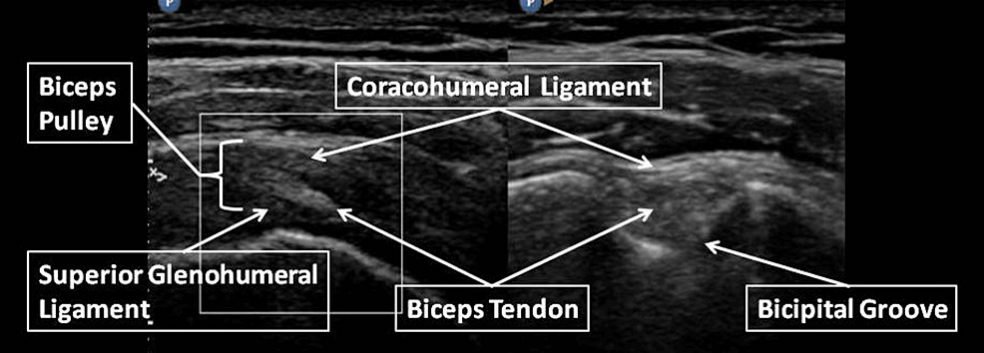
Anatomy of shoulder joint seen on ultrasound Image source: Ref. [8].
